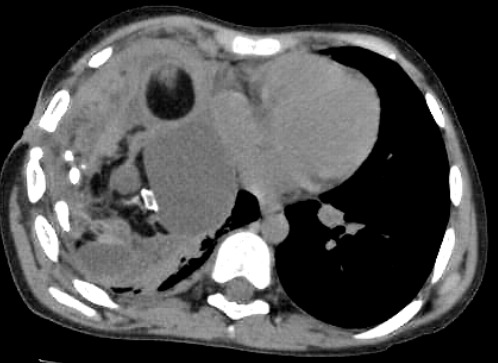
Figure 2: CT scan of thorax shows calcified lesions in a cyst in the mediastinum suggestive of bone and teeth in the mass.
